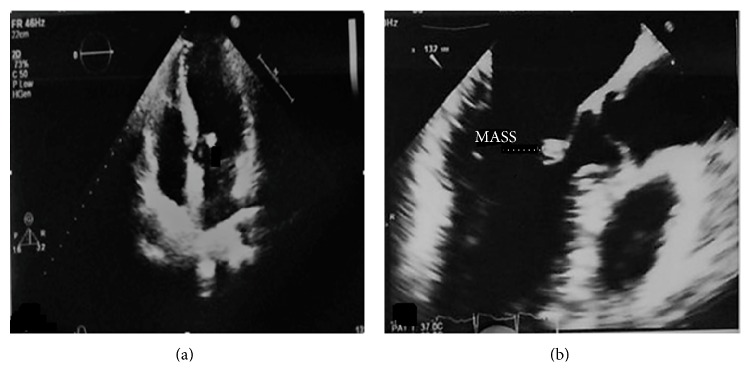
Transesophageal echocardiography showing an echogenic, round, and mobile mass, attached to the atrial surface of the anterior mitral valve leaflet by a short pedicle, measuring 5 × 5 mm.
